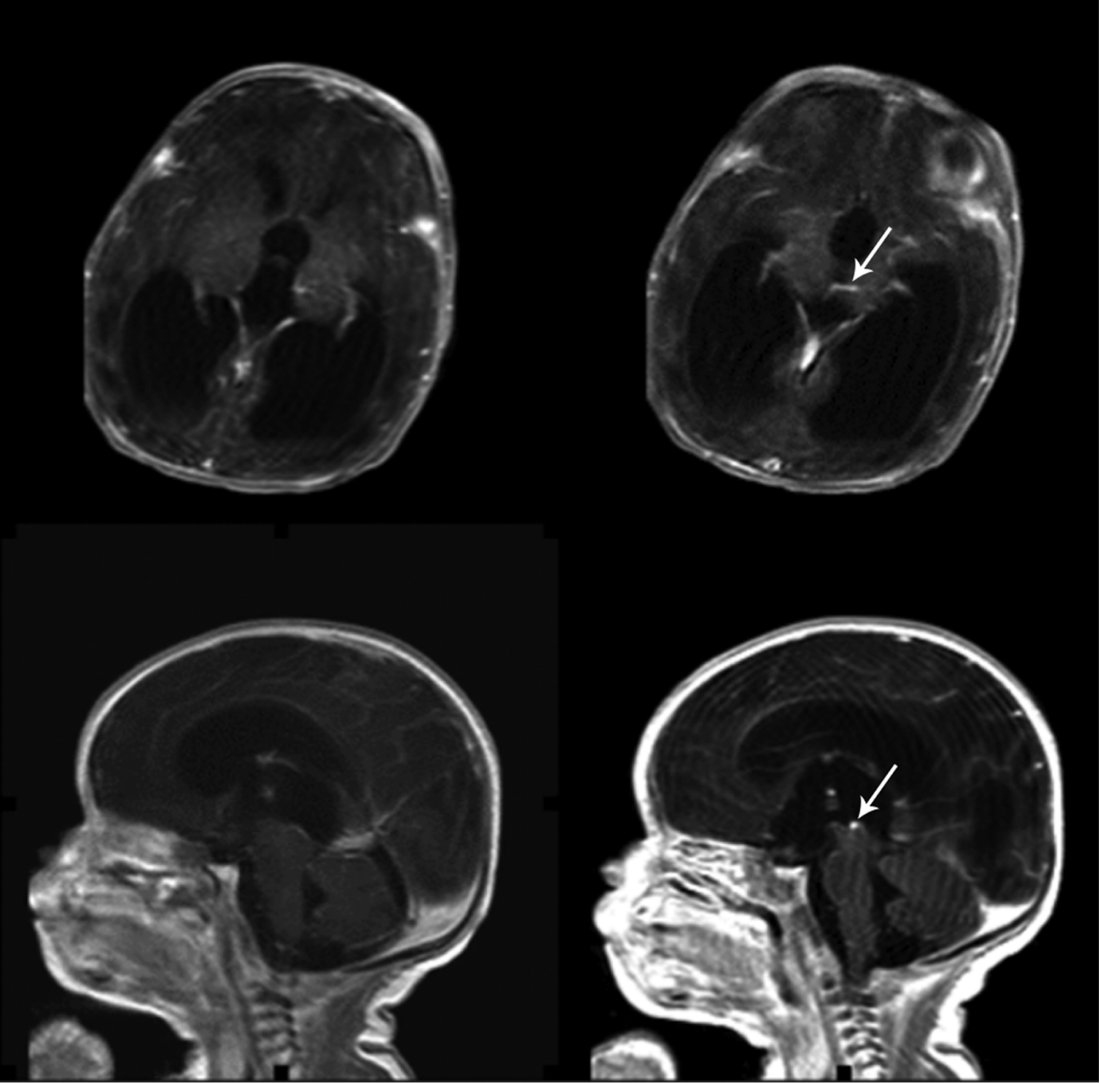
Postnatal T1-weighted axial and sagittal MRI with gadolinium at three days of life demonstrates a patent aqueduct of Sylvius, but a developmental venous anomaly (white arrow) extending from the left thalamus converging on a central draining vein bridges across the proximal orifice of the aqueduct of Sylvius and obstructs the aqueduct.
